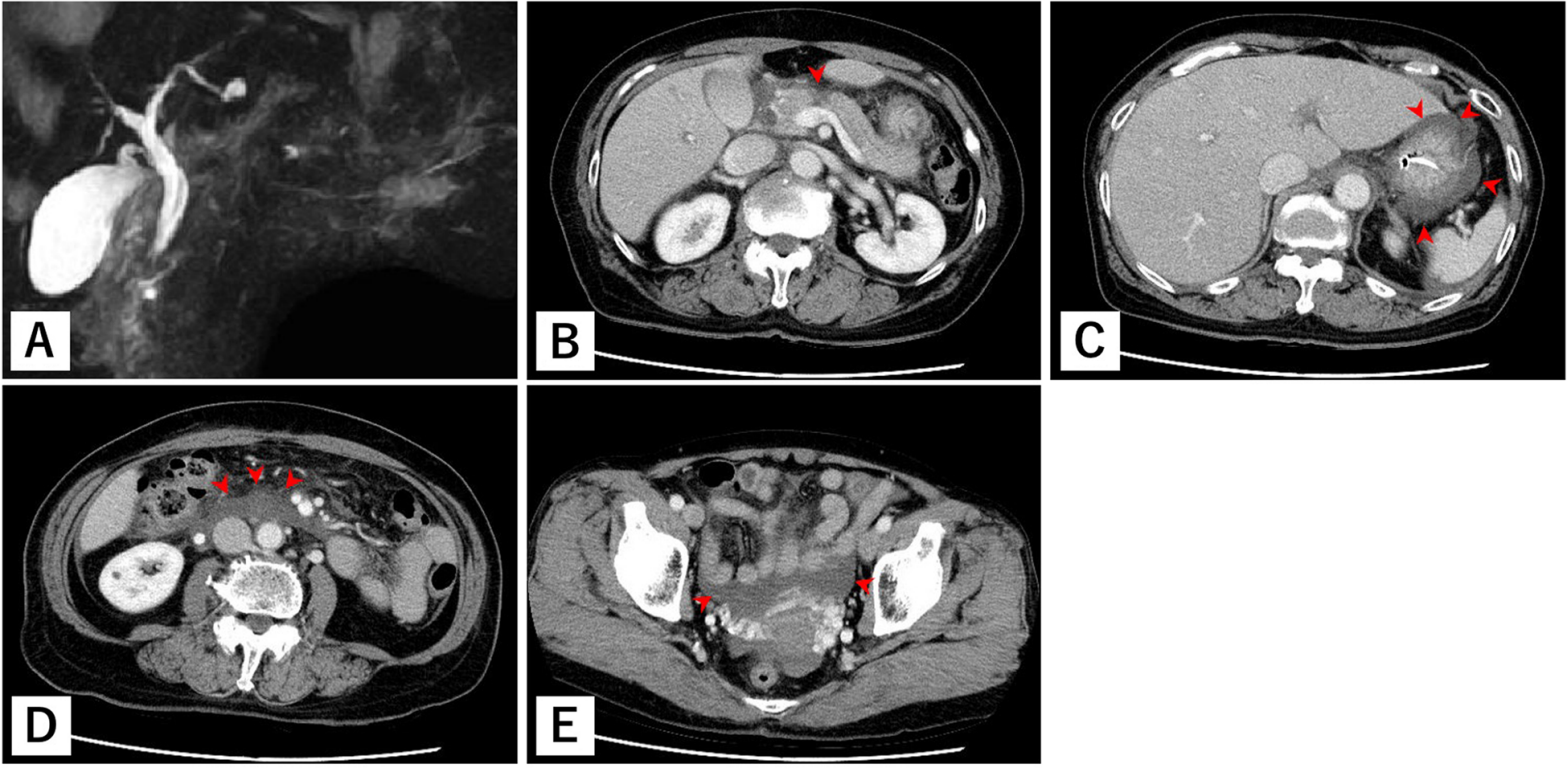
Preoperative MRCP and CT findings. From MRCP, MPD disruption was unidentified (a). The CT scan revealed pancreatic injury (b) and increase in fluid collection around the stomach (c), the pancreatic injury site, retroperitoneum (d), and pelvic cavity (e)
